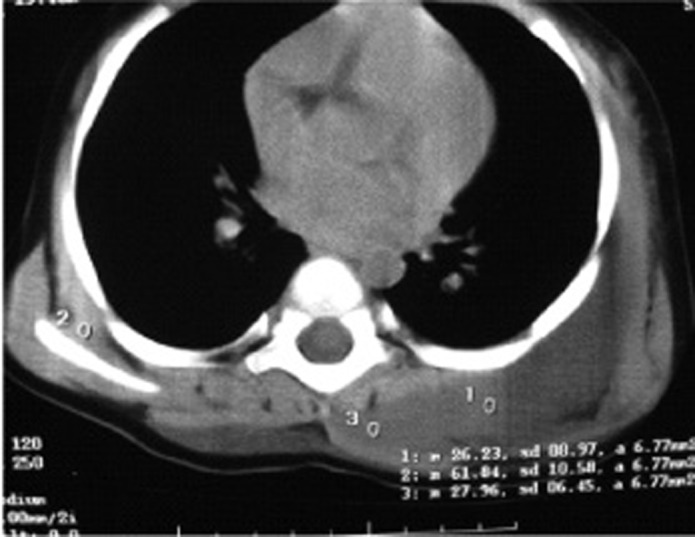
Thoracic CT-scan of this patient indicating soft tissue masses, 25 HU in density without bone formation and calcification in the prescapular and paravertebral regions. CT scan of the patient before calcification shows soft tissue masses in the posterior scapula region, prominent on the left side and a paraspinal soft tissue mass in the left upper thoracic region
